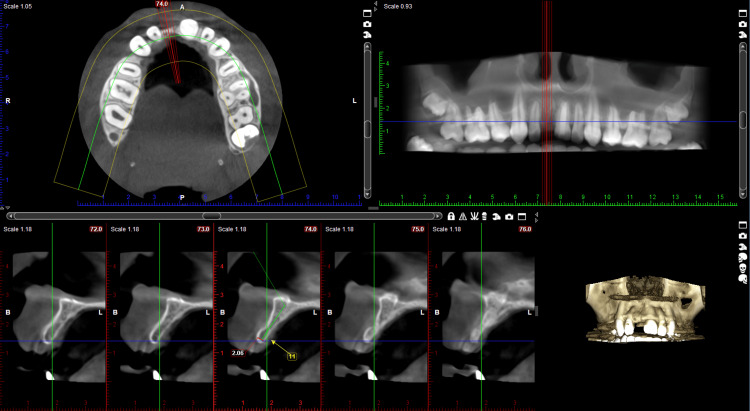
Preoperative CBCT showing missing tooth in the 11th region CBCT: Cone beam computed tomography Available buccolingual dimensions were 2.06 mm, and the apico-coronal dimension was 13 mm
